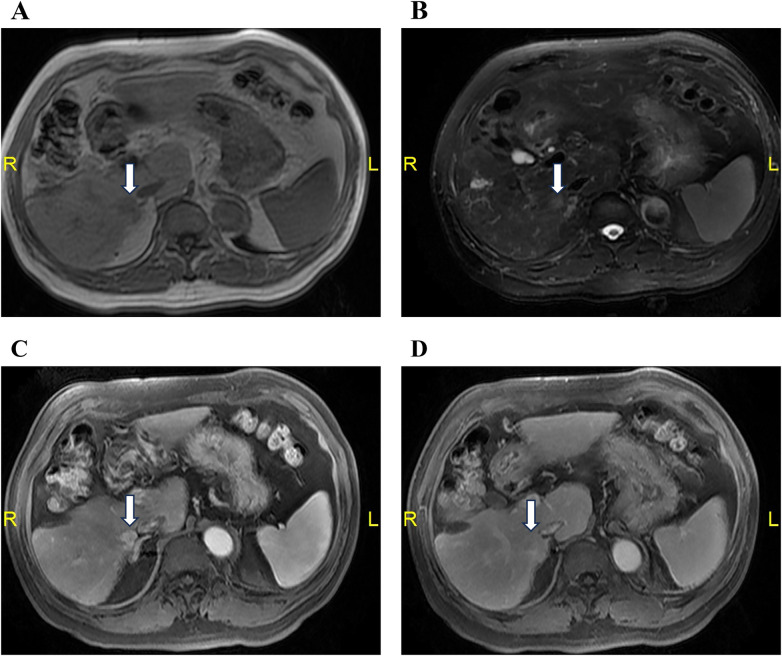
Contrast-enhanced MRI revealed a recurrent lesion in segment 6 of the liver, located superior to the right adrenal gland and to the right of the inferior vena cava. The lesion is hypointense on T1WI (A), hyperintense on T2WI (B), with arterial phase hyperenhancement (C) and portal venous phase washout (D).
